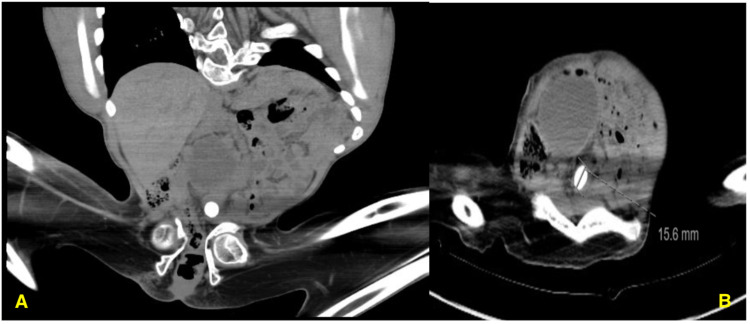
(A) Coronal view of a preoperative CT scan showing a 15.6 mm distal ureteric stone and a pelvic kidney; (B) axial view of a preoperative CT scan showing a 15.6 mm distal ureteric stone and a pelvic kidney with hydronephrosis
